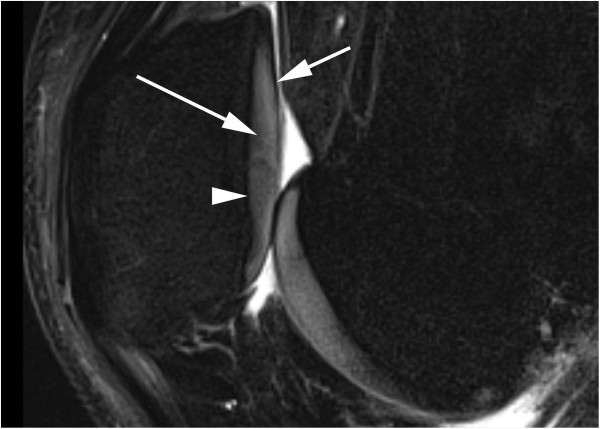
Magnetic resonance image of normal articular cartilage. Sagittal fat
